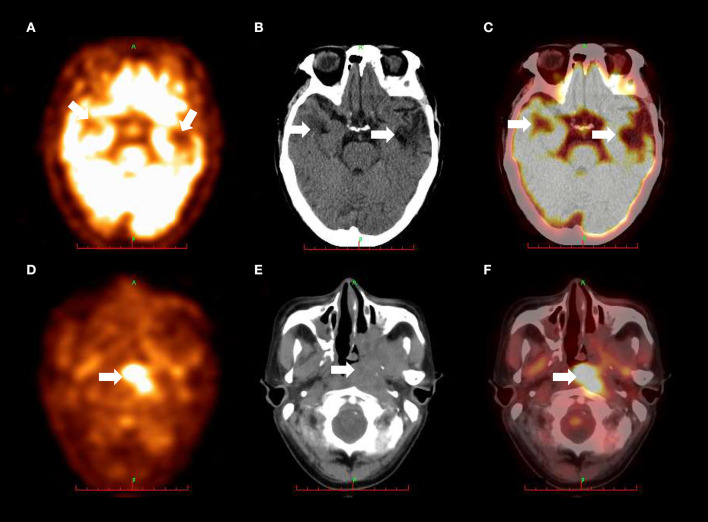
(A) PET scan revealed decreased *fluorodeoxyglucose* uptake in the bilateral temporal lobes (arrows). (B) CT scan showed patchy low-density areas in the bilateral temporal lobes (arrows). (C)
